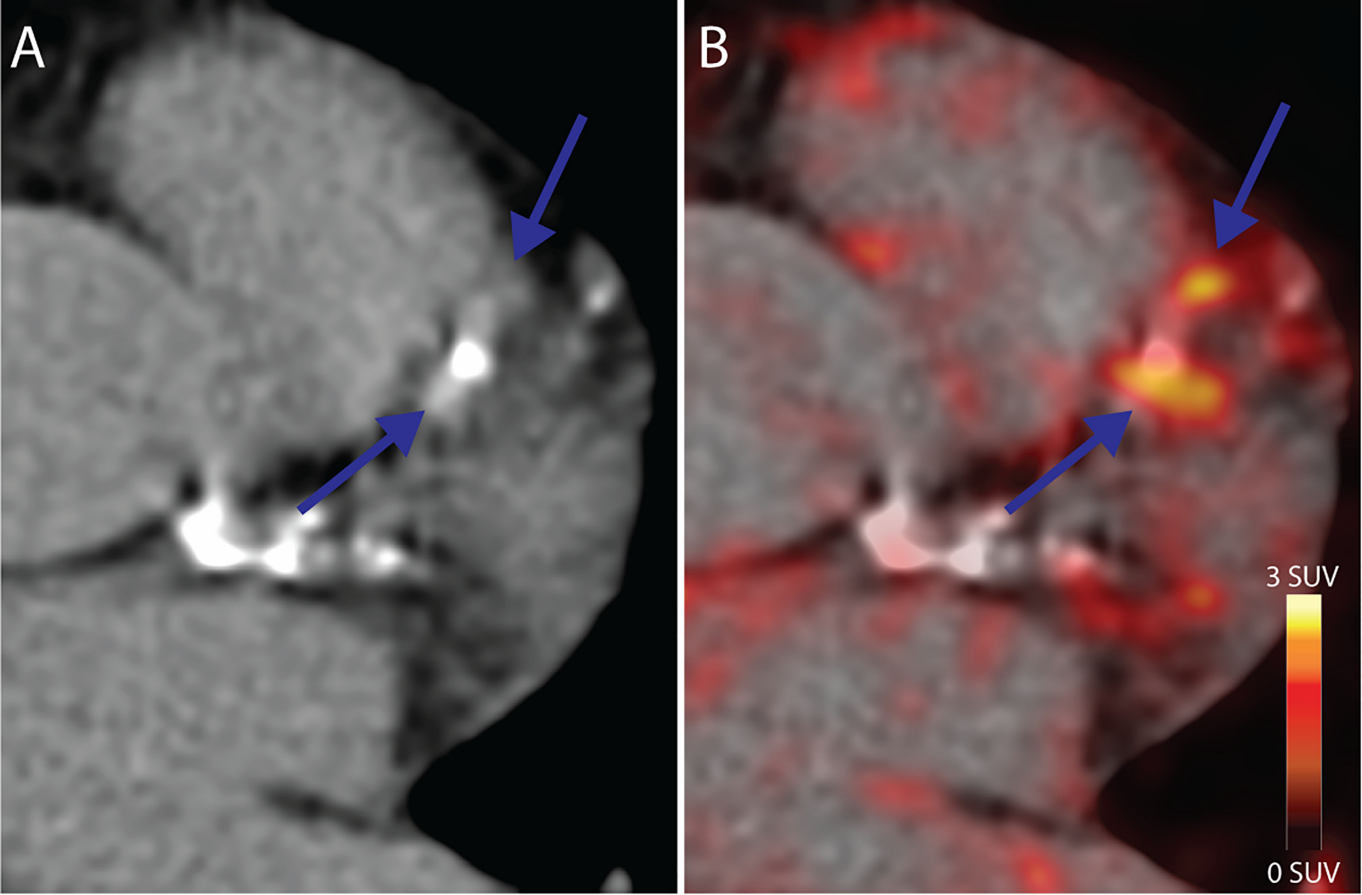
Coronary PET/CT [^64^Cu]Cu-DOTATATE imaging. (A) CT Image from a 64-year old male at baseline showing calcified lesions of the left anterior descendant artery with focal (B) [^64^Cu]Cu-DOTATATE uptake on PET (blue arrows) bordering the calcified lesions. CT, computed tomography; PET, positron emission tomography.
